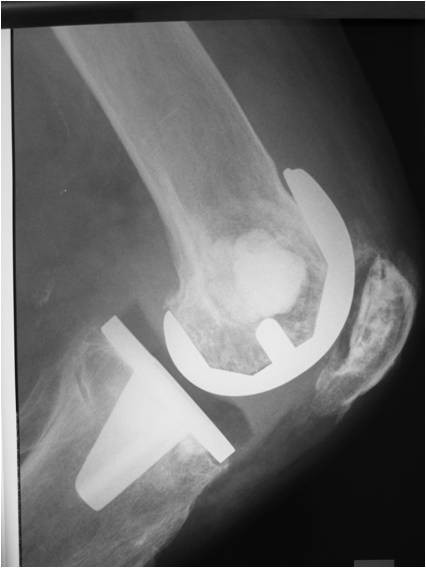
Lateral radiograph of the knee demonstrating the area of calcium phosphate bone cementage in the medial femoral and medial tibial condyles.
